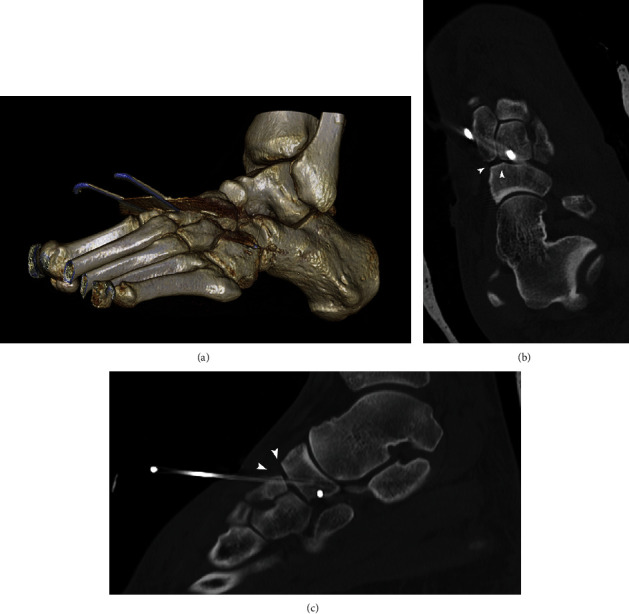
(a) Dorsolateral volume-rendered CT of the left foot shows the incomplete reduction of the calcaneocuboid joint. (b) Oblique and (c) lateral multiplanar reformatted CT show the incomplete naviculocuneiform reduction (white arrowheads).
